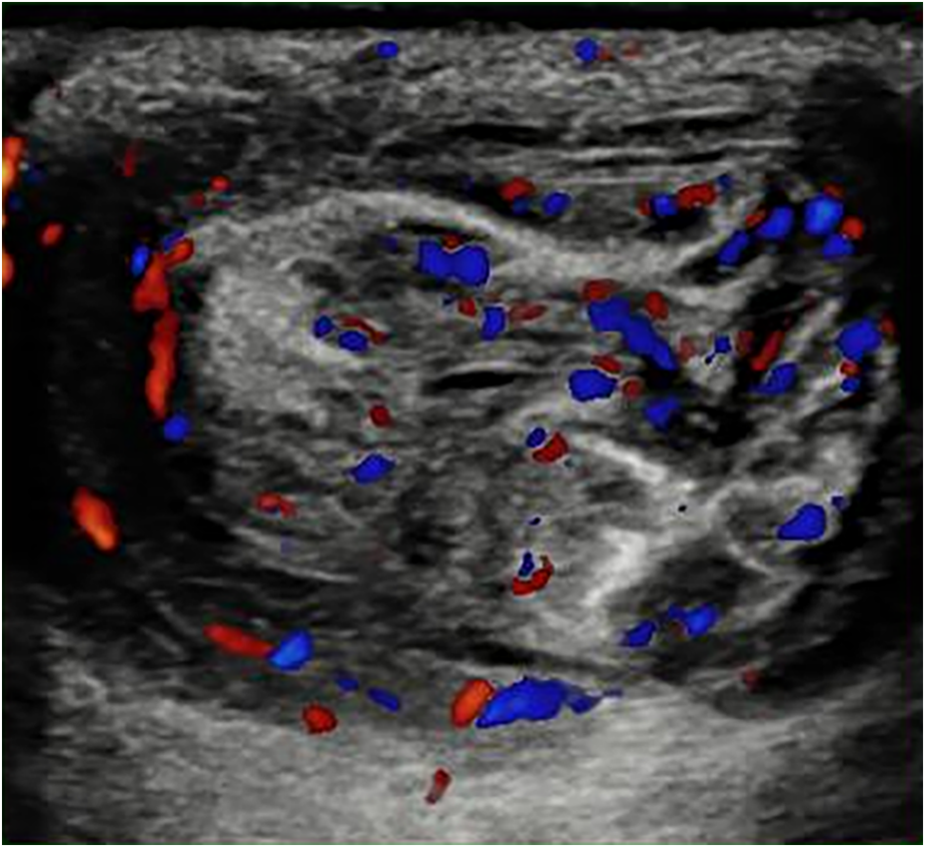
Abdominal ultrasound executed in the emergency department showing an herniated loop at umbilical site with no vascular abnormality signals on Doppler at patient presentation.
